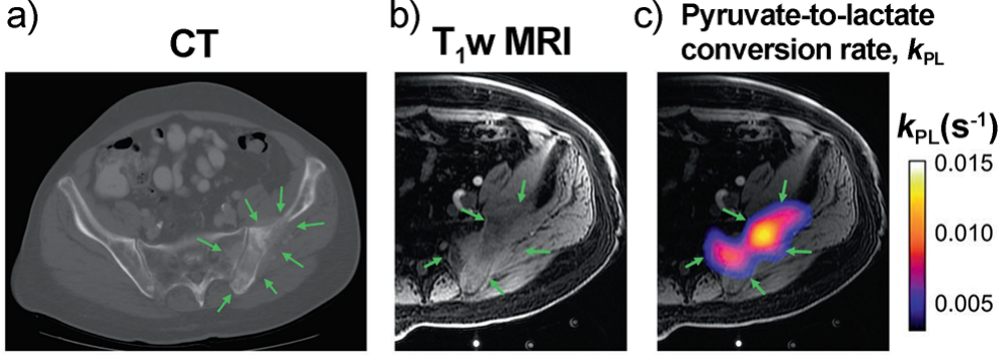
(a) A CT image of a patient with metastatic
